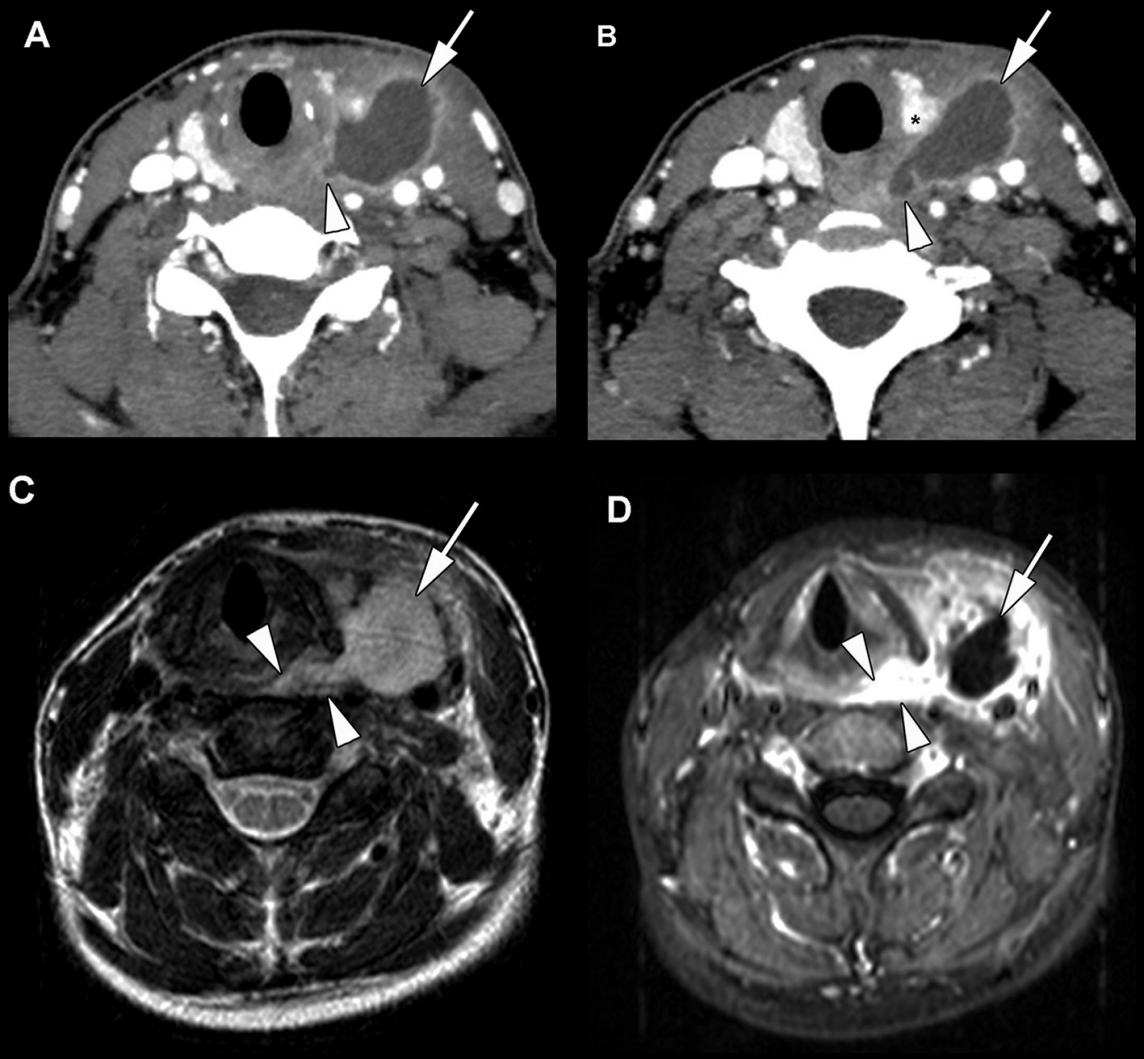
Nineteen year old patient, presenting in the emergency department with fever, throat pain, and left side neck swelling. The patient experienced three similar episodes during the last 3 years. (A,B) Contrast-enhanced CT study, obtained at admission to the emergency department. Hypodense mass lesion with rim enhancement (arrow) between the left thyroid lobe (X) and neck vessels, extending to the region of the left pyriform sinus apex and esophageal verge (arrowhead). These findings are compatible with an abscess secondary to a fourth branchial arch fistula. After abscess drainage and intravenous antibiotics, direct laryngoscopy confirmed the presence of a fistula originating from the left pyriform sinus. After endoscopic resection of the fistula, the patient remained symptom free. (C,D) MRI study, performed in another hospital, 3 years earlier. T2-weighted (C) and gadolinium-enhanced T1-weighted (D) image show similar findings as in images (A,B) collection (arrow) with surrounding inflammation, connecting to the pyriform sinus (arrowheads). This was recognized as a neck abscess, but the diagnosis of a fourth branchial arch anomaly was not made. Treatment at that time was abscess drainage and intravenous antibiotics.
